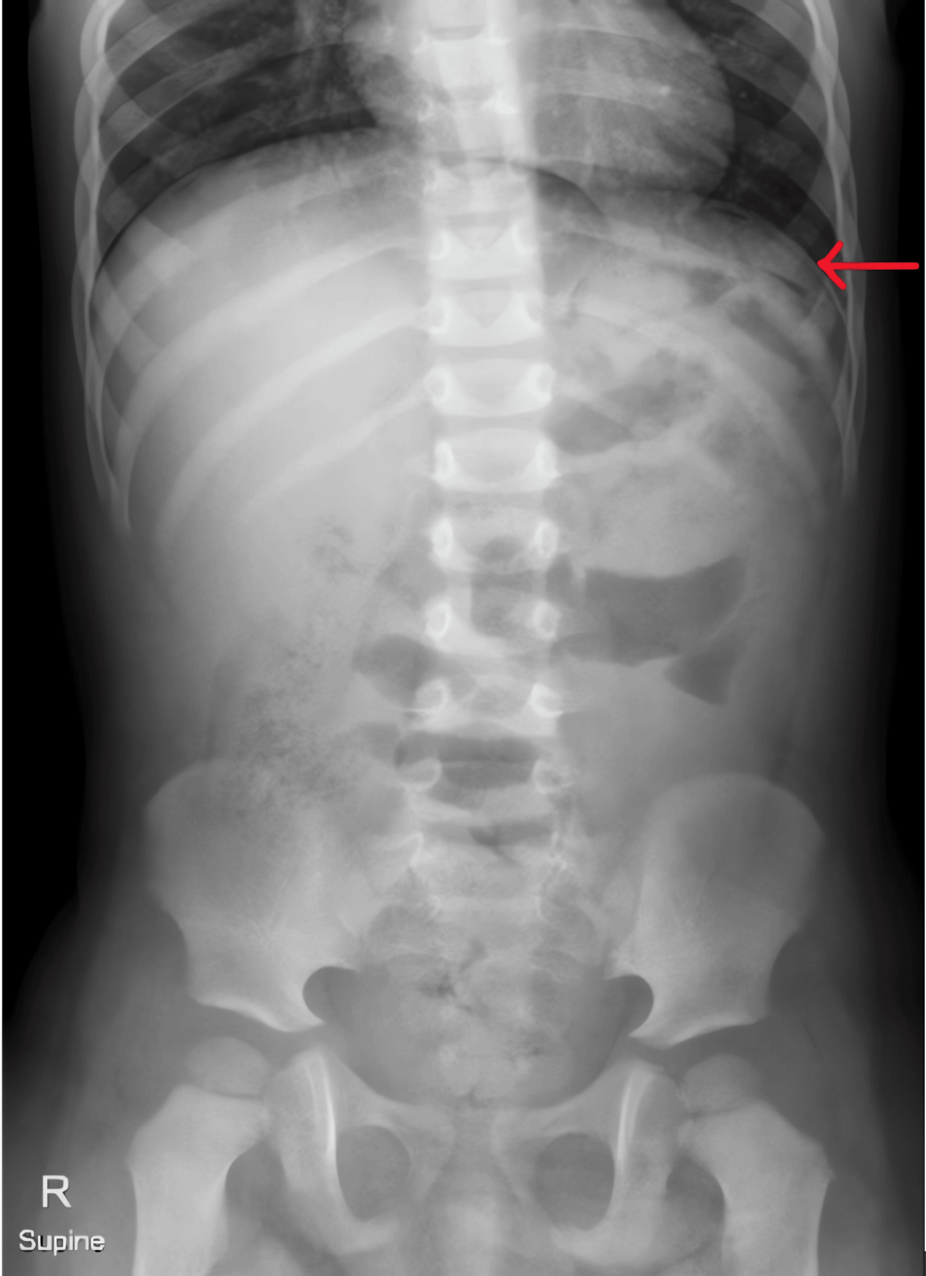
Erect abdominal radiograph showing pneumoperitoneum Subdiaphragmatic free air is evident, suggesting a perforation of a hollow viscus.
